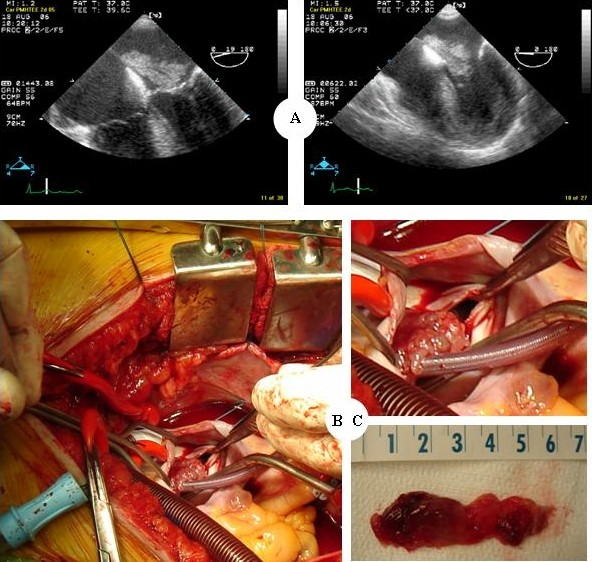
Transesophageal echocardiography shows a mobile mass in the left atrium, which does not obstruct the mitral valve. (A), After performing cardiopulmonary bypass, the retractor allows visualization of the left atrium, and the atrium is then opened by a blade. The myxoma is attached from the atrial septum. (B), LA myxoma: tan and jelly-like tissue with an aggregate measurement of 6 cm × 1.5 cm. (C).
